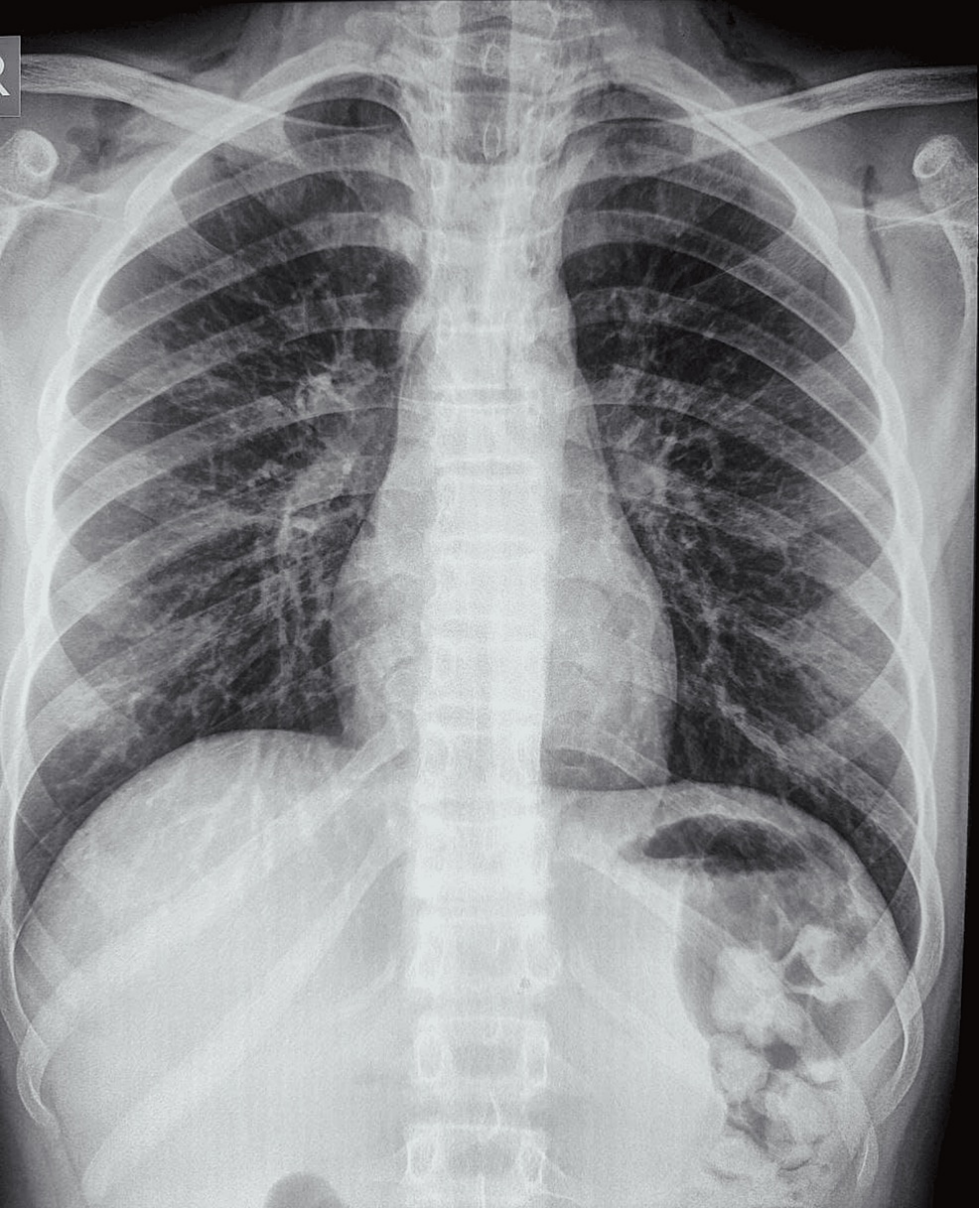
Post-rigid bronchoscopy chest radiograph demonstrating the successful retrieval of the board pin
